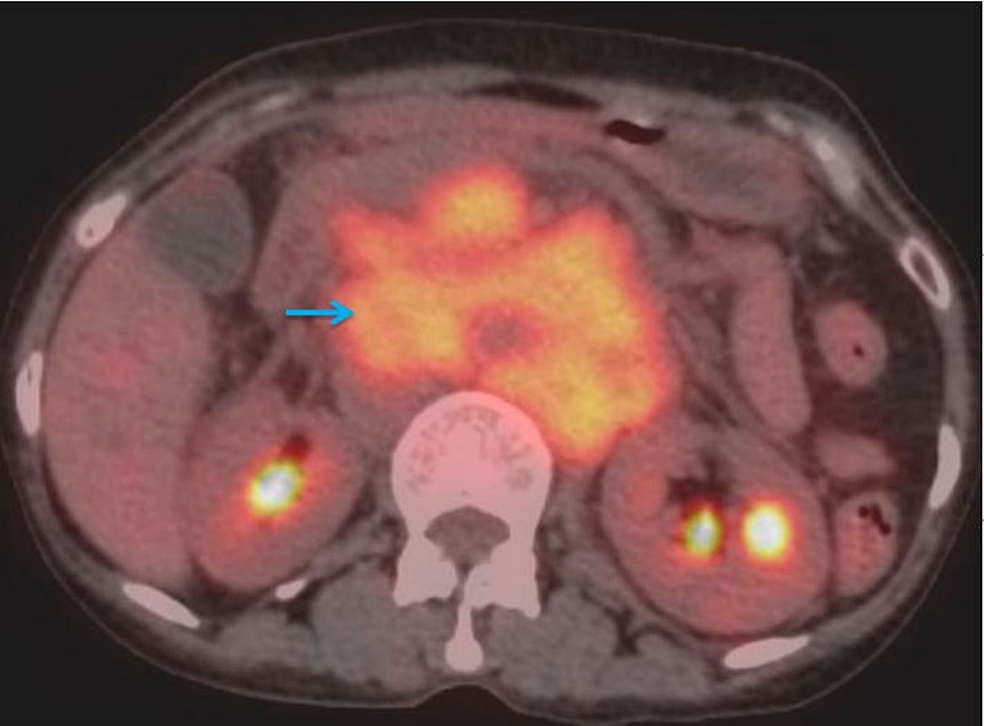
Positron emission tomography-computed tomography (axial view) showing metabolically active, large, conglomerated multiple lymph nodal mass (arrow).
